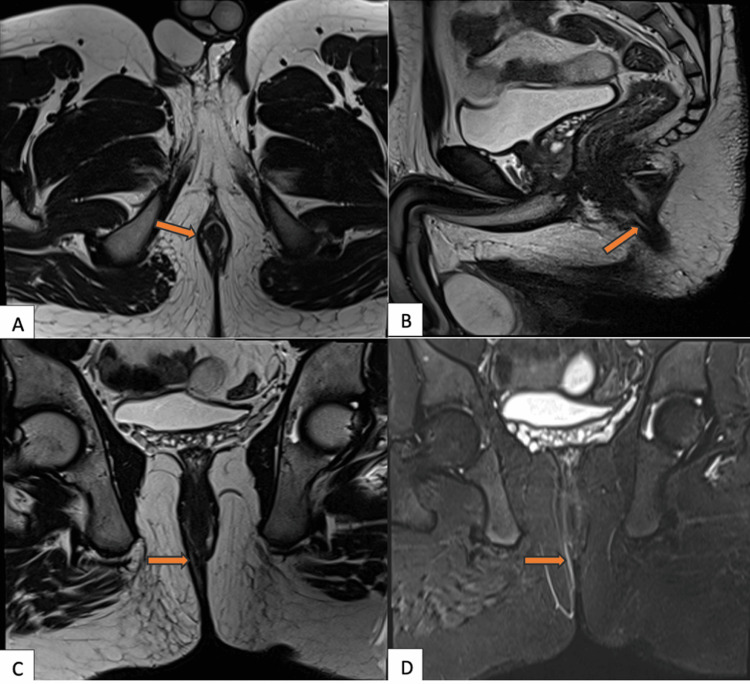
MRI fistulogram demonstrating a St. James grade 1 perianal fistula MRI: magnetic resonance imaging; STIR: short tau inversion recovery Axial (A), sagittal (B), coronal (C) T2-weighted images and coronal STIR (D) image showing a hyperintense linear tract confined to the intersphincteric space (orange arrows) without evidence of abscess or secondary tracts, consistent with a simple intersphincteric fistula
